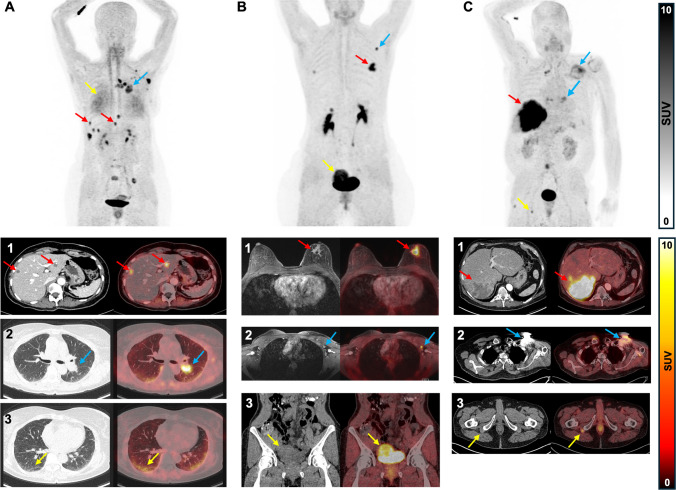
A 48-year-old female patient with metastatic breast cancer (A). Arterial-phase contrast-enhanced abdominal CT demonstrated hepatic (A1) and non-contrast enhanced CT demonstrated pulmonary metastases (A2) with intense fibroblast activation (red arrows, SUV_max_ up to 12.7; blue arrows, SUV_max_ up to 10.7). Increased fibroblast activation was also observed in fibrotic lung tissue (A3, yellow arrows, SUV_max_ up to 5.4). A 45-year-old female patient with breast cancer and lymph node metastasis (B). T1-weighted fat-suppressed MRI revealed a primary tumour in the right breast (B1) and a solitary lymph node metastasis in the left axillary region (B2), both showing intense contrast enhancement and fibroblast activation (red arrows, SUV_max_ 13.6; blue arrows, SUV_max_ 9.7). In premenopausal women, intense fibroblast activation of the uterus represents a physiological finding, as demonstrated on coronal non-contrast-enhanced CT (B3, yellow arrows, SUV_max_ up to 9.8). A 72-year-old male patient with cholangiocellular carcinoma (C). Arterial-phase contrast-enhanced CT revealed a large lesion in the right hepatic lobe with marginal arterial hyper perfusion and intense fibroblast activation (C1, red arrows, SUV_max_ 31.6). An implanted cardioverter-defibrillator due to dilated cardiomyopathy showed diffuse fibroblast activation, likely related to an immunogenic foreign material reaction (C2, blue arrows, SUV_max_ 9.2), as well as increased fibroblast activation in the basal left ventricular myocardium, probably reflecting fibrosis (blue arrows, SUV_max_ up to 5.6). Additionally, focal fibroblast activation was observed in the tendon of the biceps femoris muscle (C3), likely related to mechanical stress or degenerative changes (yellow arrows, SUV_max_ 7.1)
